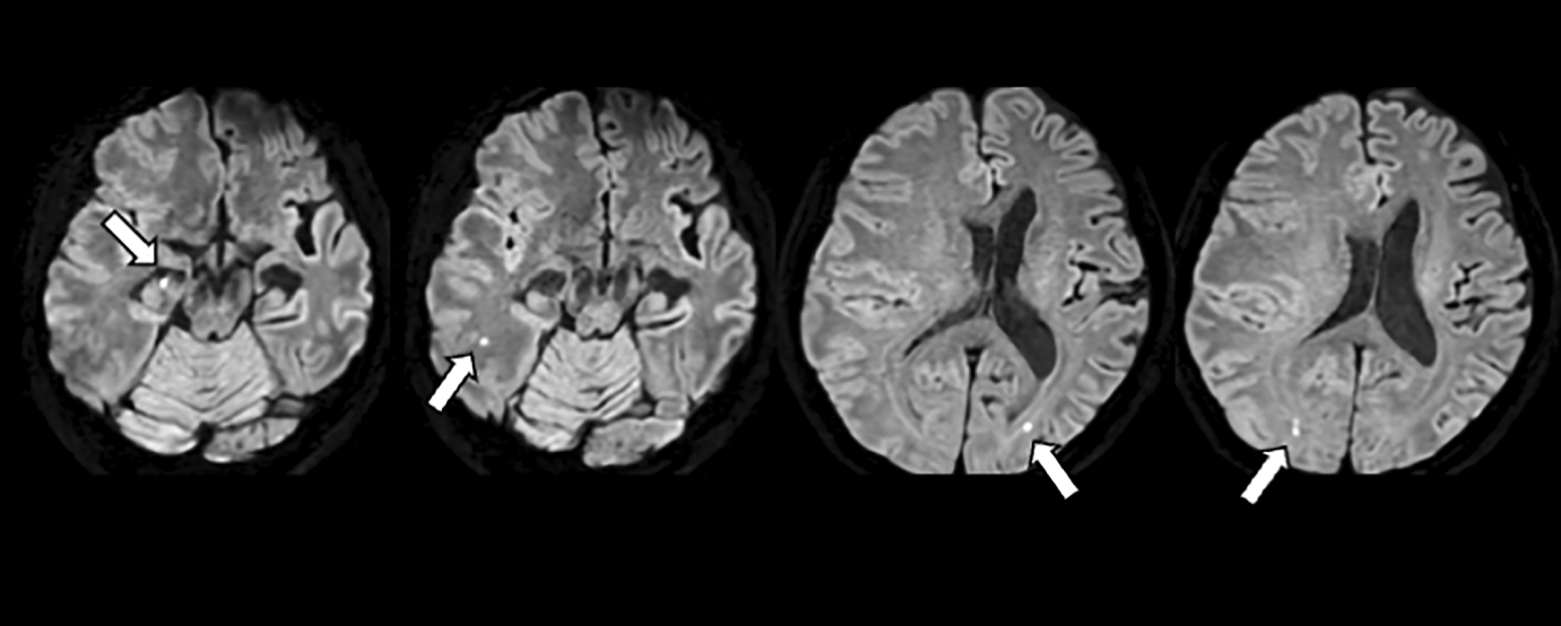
Multiple microinfarctions following lecanemab treatment. Thin‐slice diffusion‐weighted image (DWI) (slice thickness = 2 mm with gaplessness) performed 6 days following symptom onset revealed numerous punctate high‐signal areas. The white arrows show microinfarctions.
